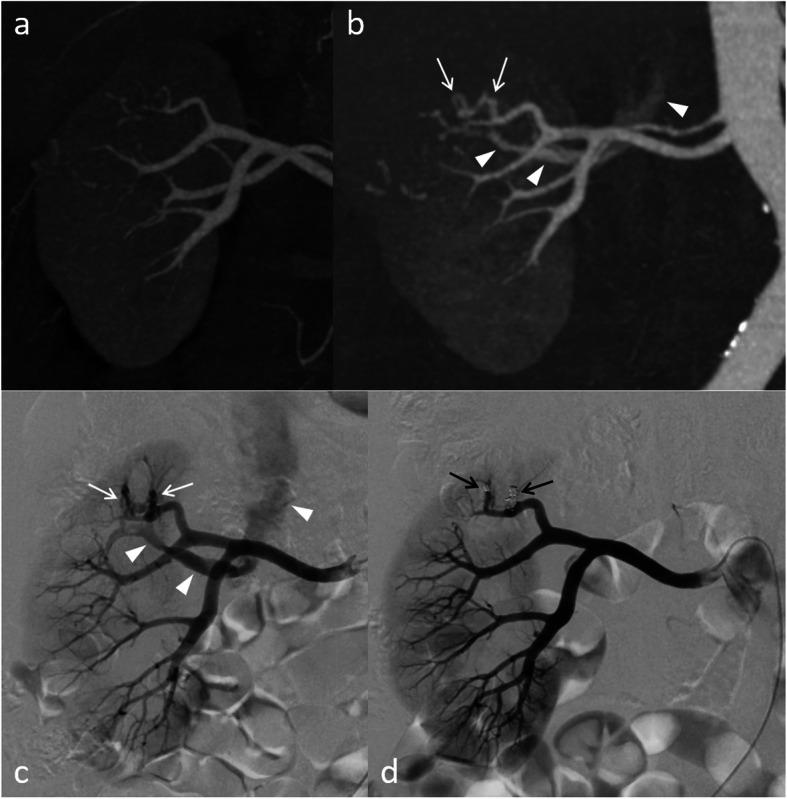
A case of two newly appearing arteriovenous fistulas (AVFs), with symptoms, in a 67-year-old woman. a No abnormal finding observed on early postoperative contrast-enhanced CT on postoperative day (POD) two. b Two AVFs (arrows) with early venous return (arrowheads) appear on CT obtained on POD 19, with hematuria after discharge. c, d Angiography showing the two AVFs (white arrows) successfully embolized using microcoils (black arrows), with early venous return (arrowheads) disappearing on POD 21
